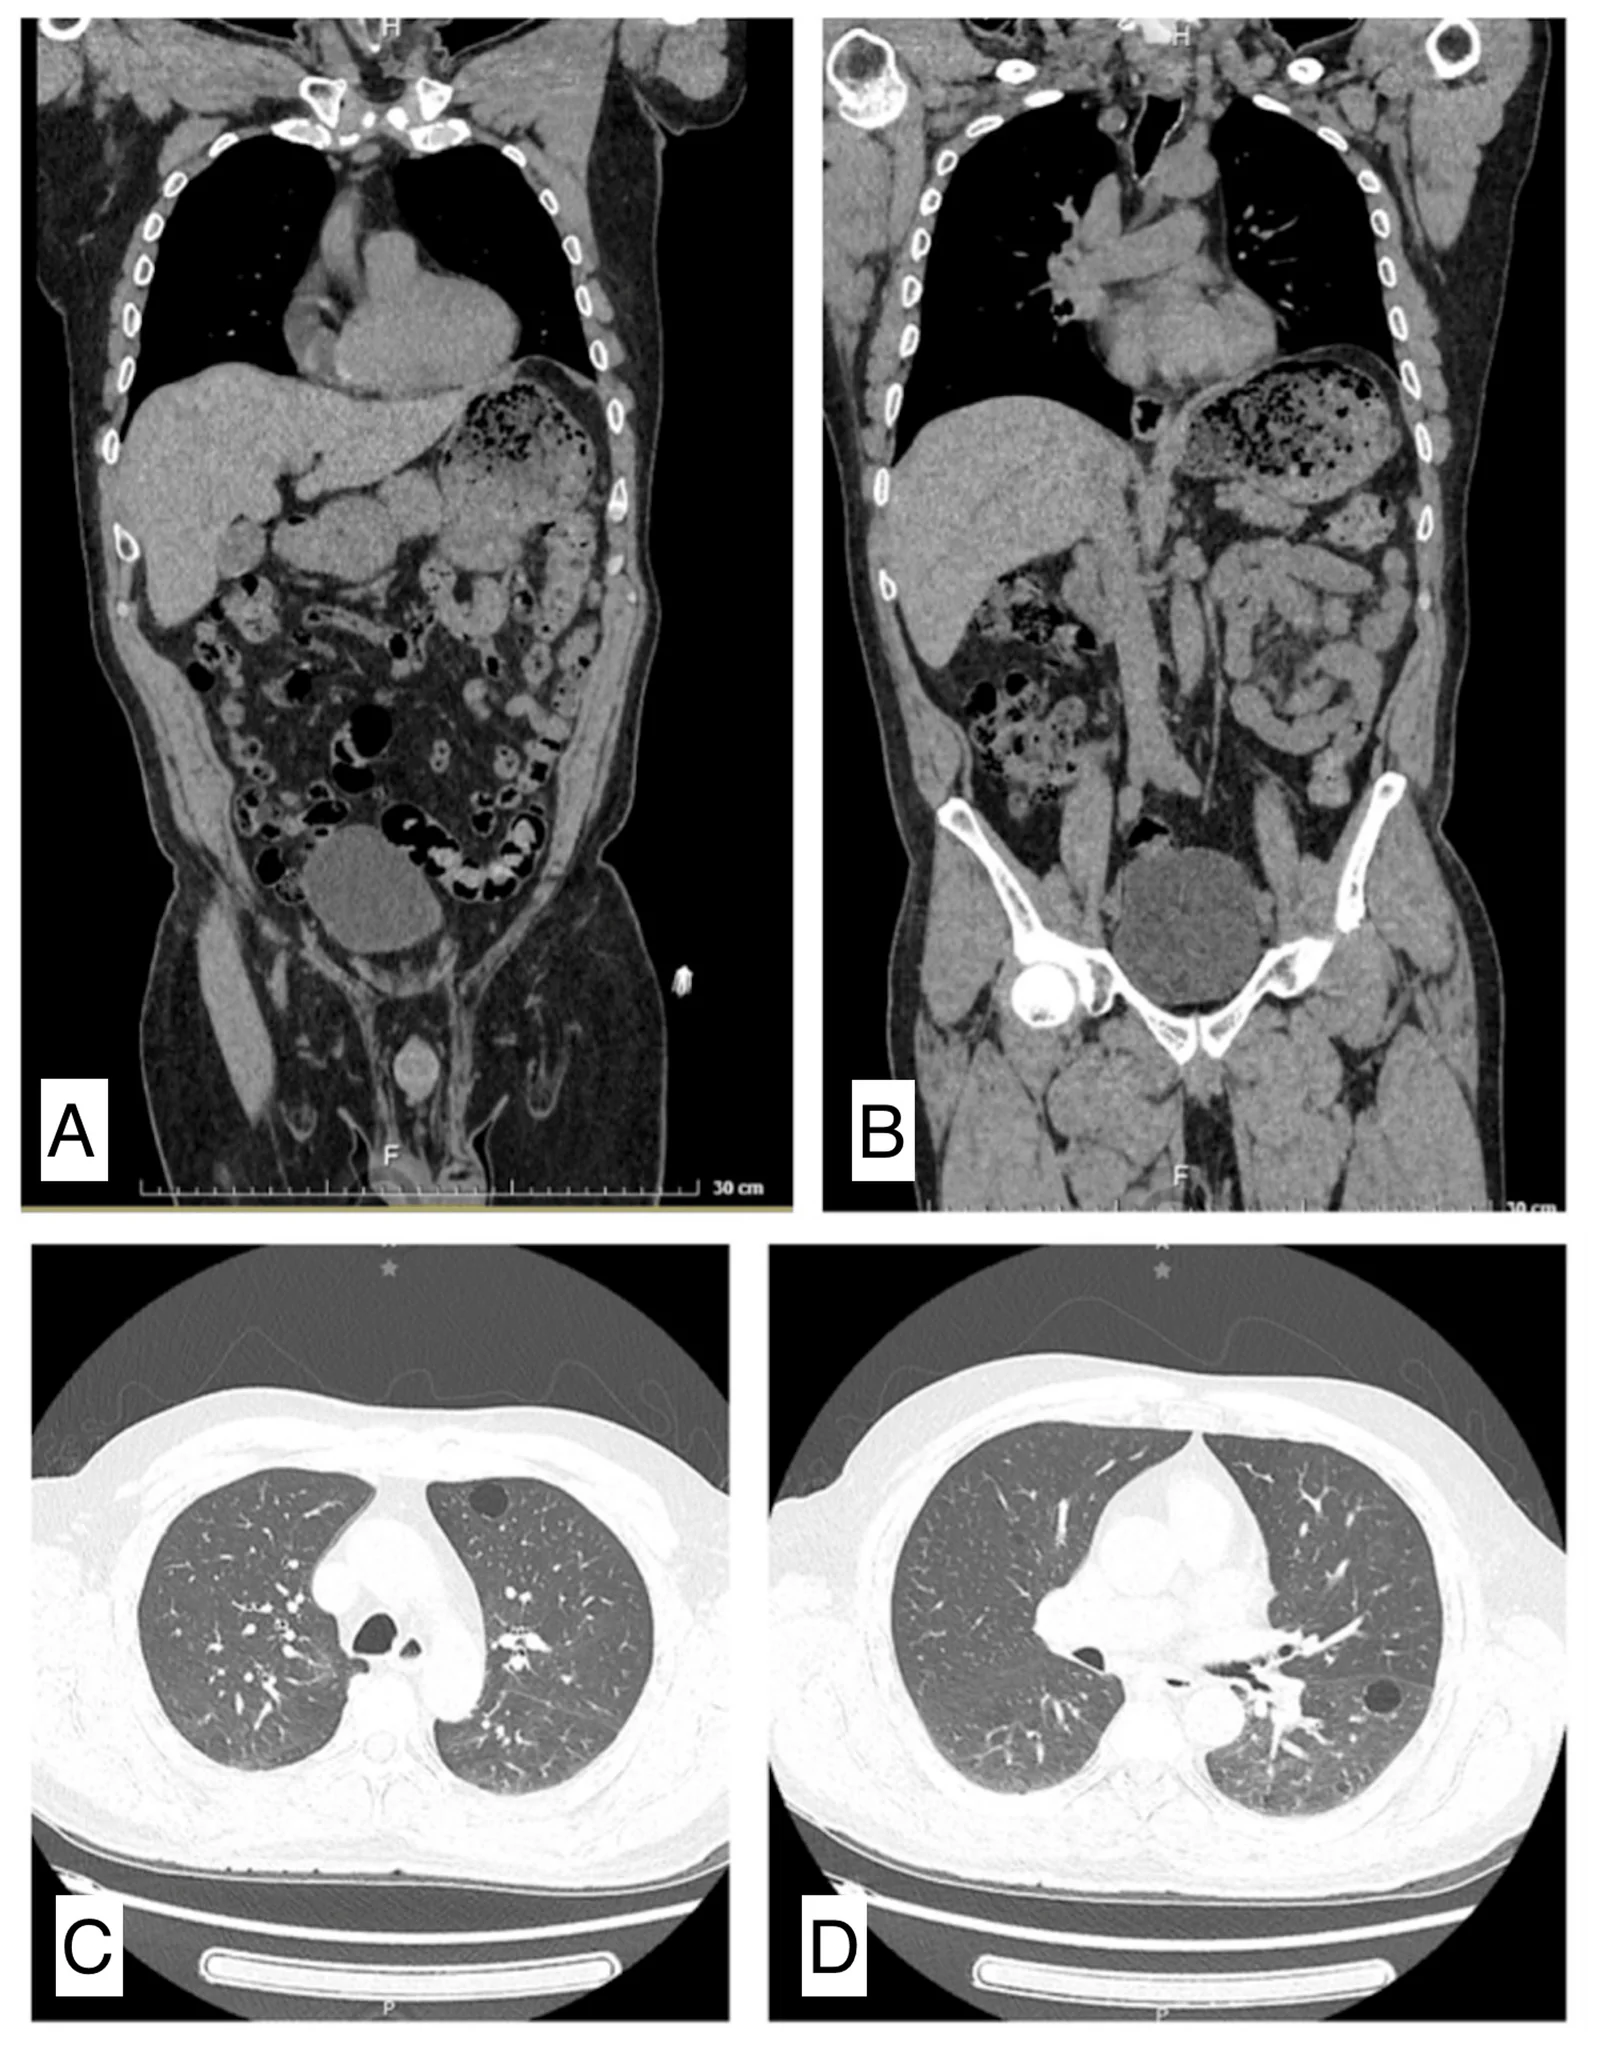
Non-contrast thoracoabdominopelvic computed tomography (A, B) Coronal reconstructions demonstrating no thoracic or abdominal findings suggestive of disseminated histoplasmosis. (C, D) Lung window images showing mild thin-walled pulmonary cysts without pulmonary nodules, consolidations, cavitary lesions, or other computed tomography findings suggestive of pulmonary histoplasmosis.
